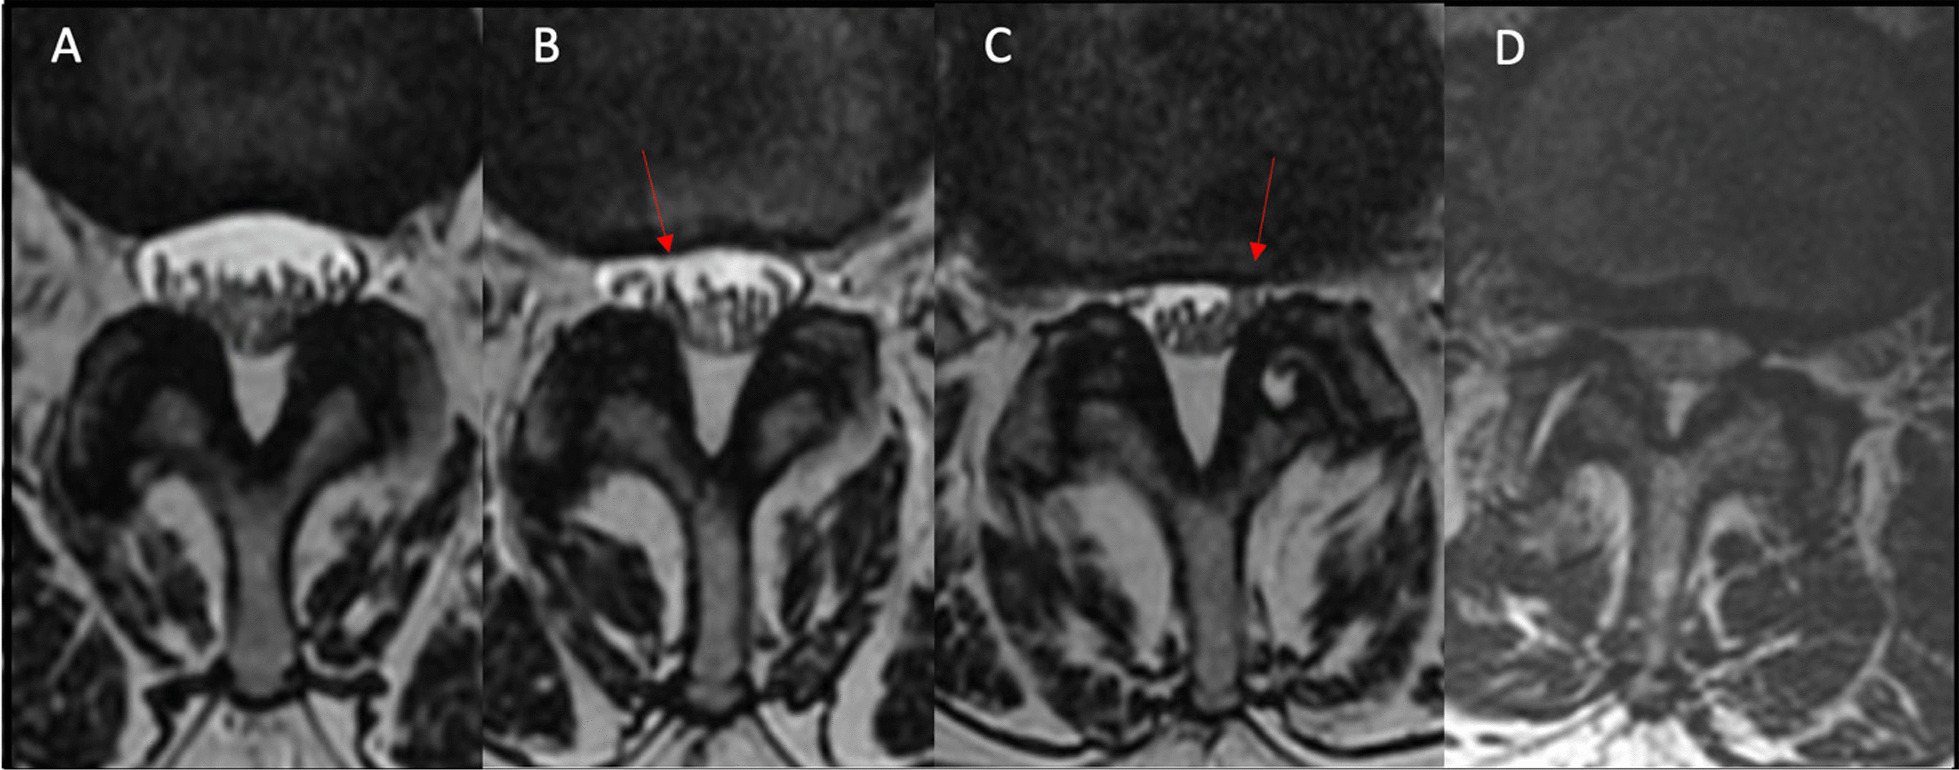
Morphological grade of stenosis [17, 20]. Axial T2-weighted MRI images shown. A Grade 0, normal thecal sac without cerebral spinal fluid (CSF) effacement. B Grade 1, mild CSF effacement without significant nerve root crowding/compression. C Grade 2, moderate CSF effacement with nerve root crowding/compression. D Grade 3, severe CSF effacement with indistinguishable individual nerve roots due to significant crowding/compression
